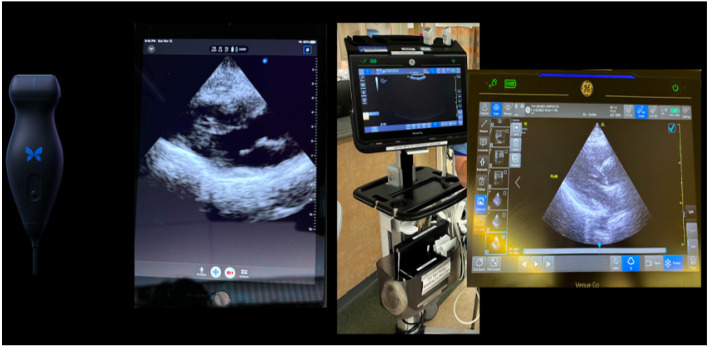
Handheld Butterfly iQ device and cart-based GE Venue Go model demonstrating parasternal long axis view.
